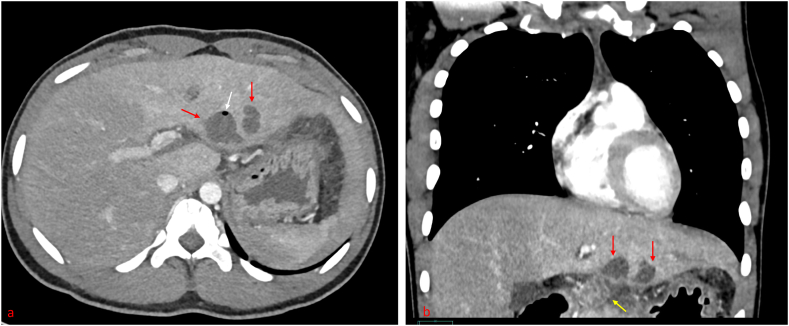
CT abdomen reflected small liver abscess.
